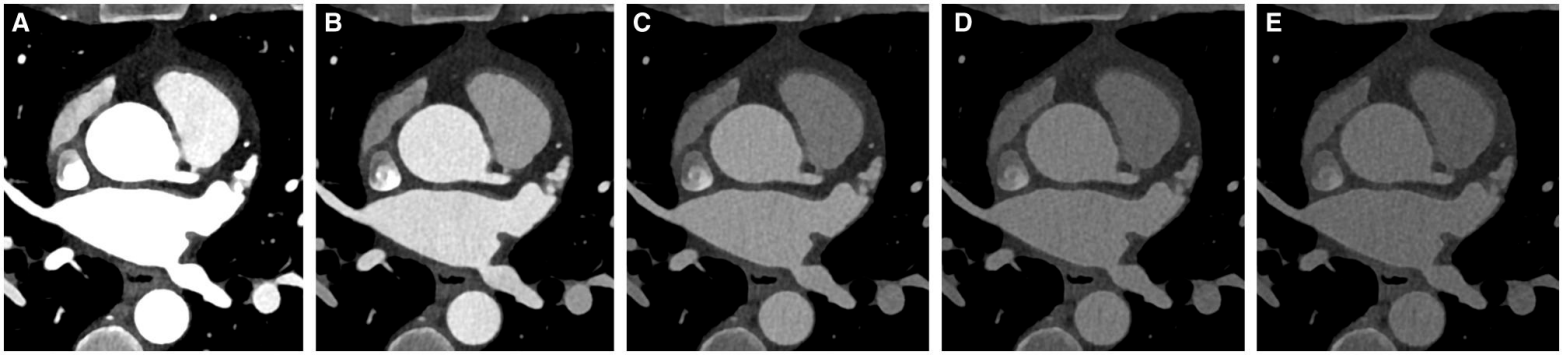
A 47-year-old female patient undergoing multienergy coronary CT angiography with a dual-source photon-counting detector CT (window width: 320; window level: 230). Axial virtual monoenergetic image reconstructions at the origin of the left main artery showing progressively decreasing contrast-to-noise (CNR) ratios at increasing monoenergetic levels, namely 40 keV (A), 60 keV (B), 80 keV (C), 100 keV (D), and 120 keV (E).
